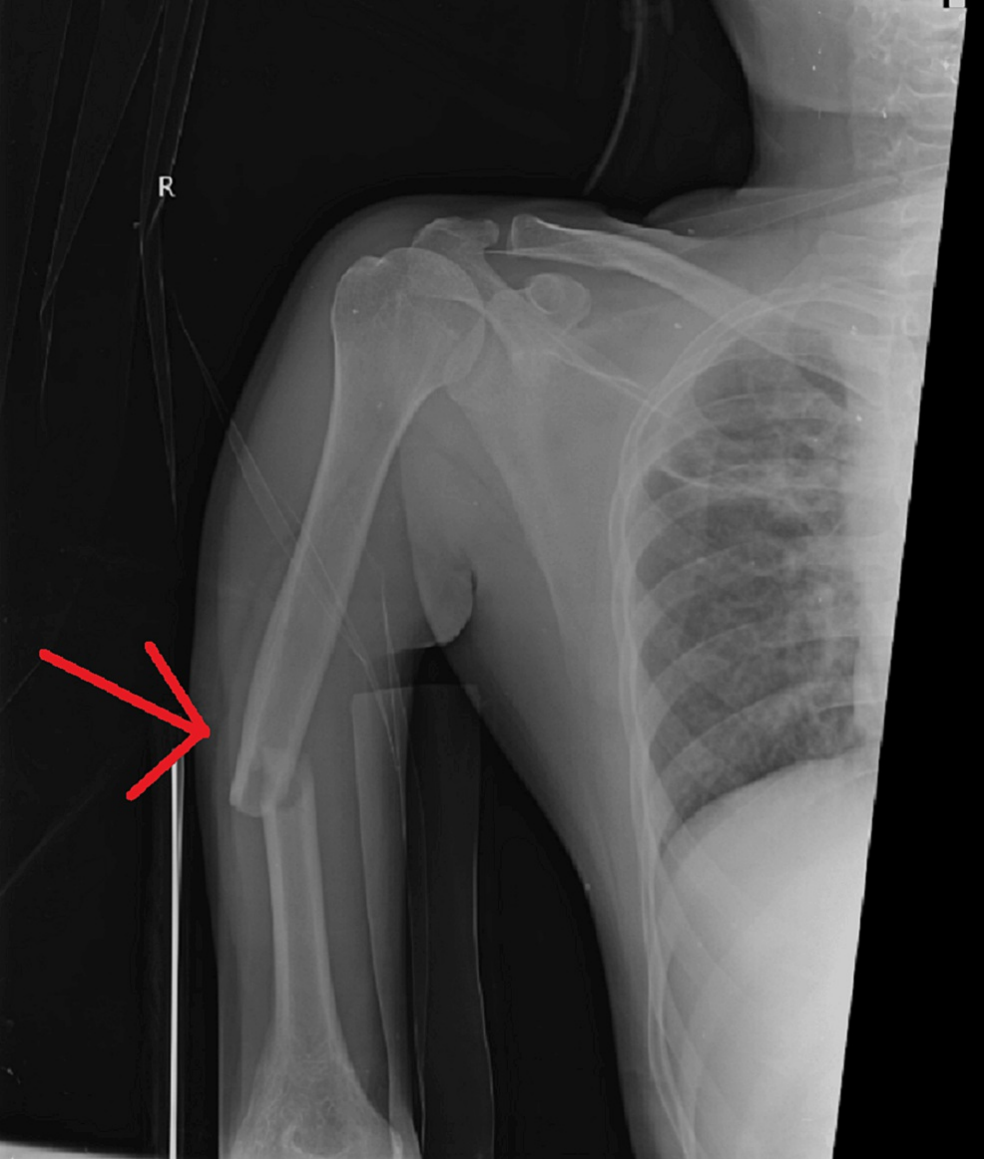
Anterior-posterior radiograph showing short oblique fracture mid-shaft region on right side
